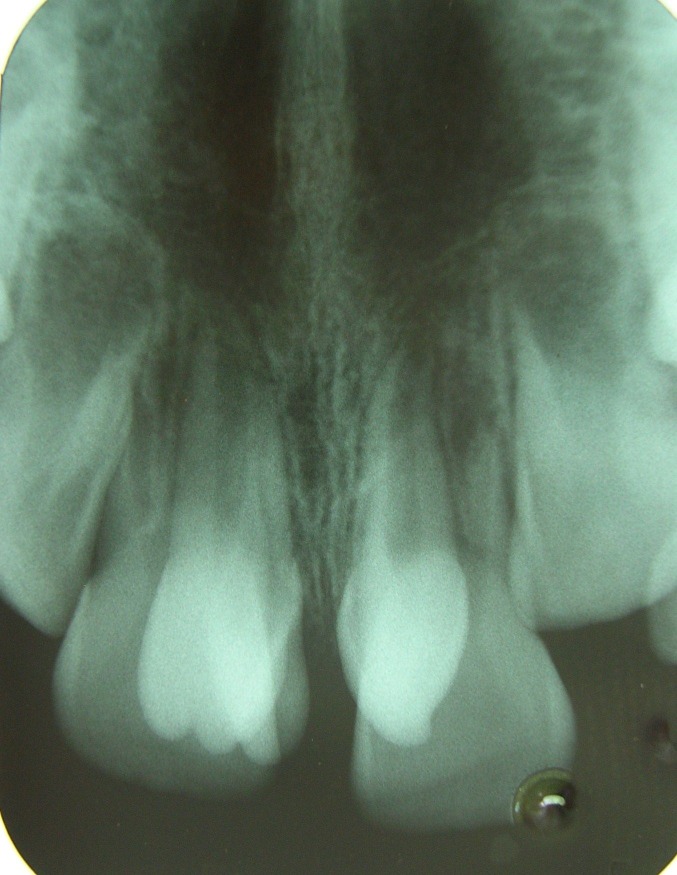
Talon cusp is an accessory cusp-like structure, which arises during the morphodifferentiation stage of tooth development. This cusp can occur on lingual or labial surfaces of either primary or permanent anterior teeth.^8,9^ Histomorphometric examination has revealed that the talon cusp has normal enamel and dentin with a substantially enlarged pulp tissue, which suggests that talon tooth may be a developmental anomaly originating in the stage of morphodifferentiation.^10^ Using a non-destructive investigation of the taloned crown undercone-beam x-ray computed tomography has revealed pulpal extensionsin two talon cusps.^11^
